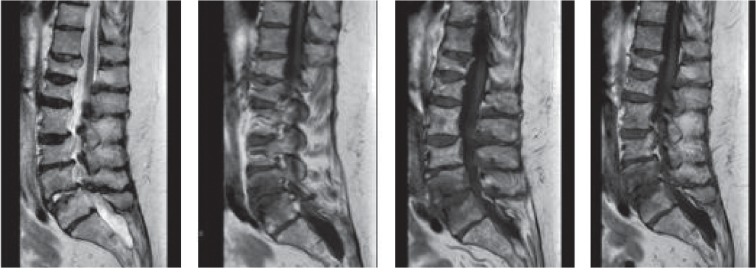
Axial and sagittal T1 and T2-weighted and coronal and sagittal short-TI inversion recovery (STIR) images of the lumbar spine without intravenous contrast administration. Axial and sagittal T1-weighted images were then acquired following intravenous administration of 80 mL of Gadavist. The image shows vertebral body edema and enhancement most evident at the L4L5 level. Paravertebral soft tissue thickening is seen at the L5-S1 level, with extension into the left neural foramina. Findings are suggestive of osteomyelitis/discitis.
